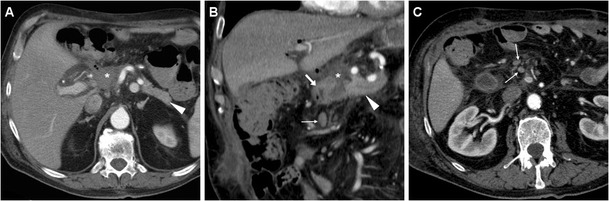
a-c Postoperative pancreatic fistula (PF) after Whipple PD for pT3N1 pancreatic ductal adenocarcinoma in a 72-year-old man with persistent output and increasing amylase levels in fluid from drainage. On postoperative day 9, CT (Fig.3) showed a mixed-attenuation collection (*) extending upwards from the PJS, between the PR (*arrowheads*) and MJL (*arrows*), consistent with clinico-biological diagnosis of PF. These CT changes ultimately resolved on conservative treatment. Note intrahepatic pneumobilia (in b), some small-sized lymph nodes (*thin arrows*) surrounding the superior mesenteric vessels. (Partially adapted with permission from Tonolini [25])
